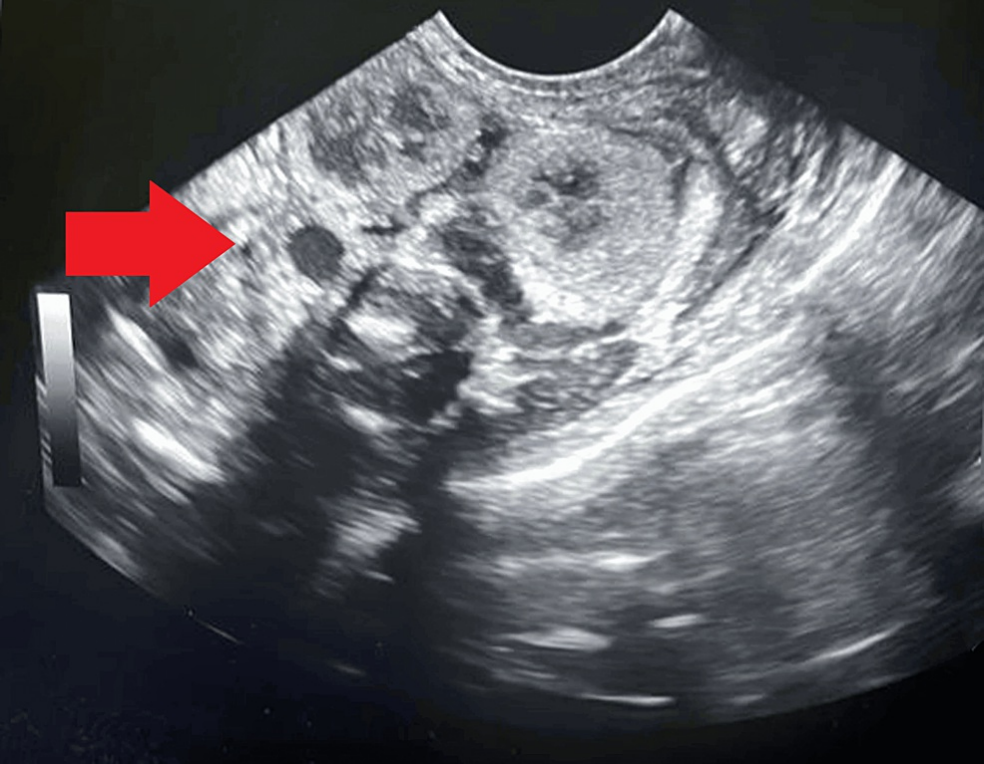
Ultrasonography revealed right-crossed renal ectopia and Grade I renal parenchymal disease with minimal ascites
